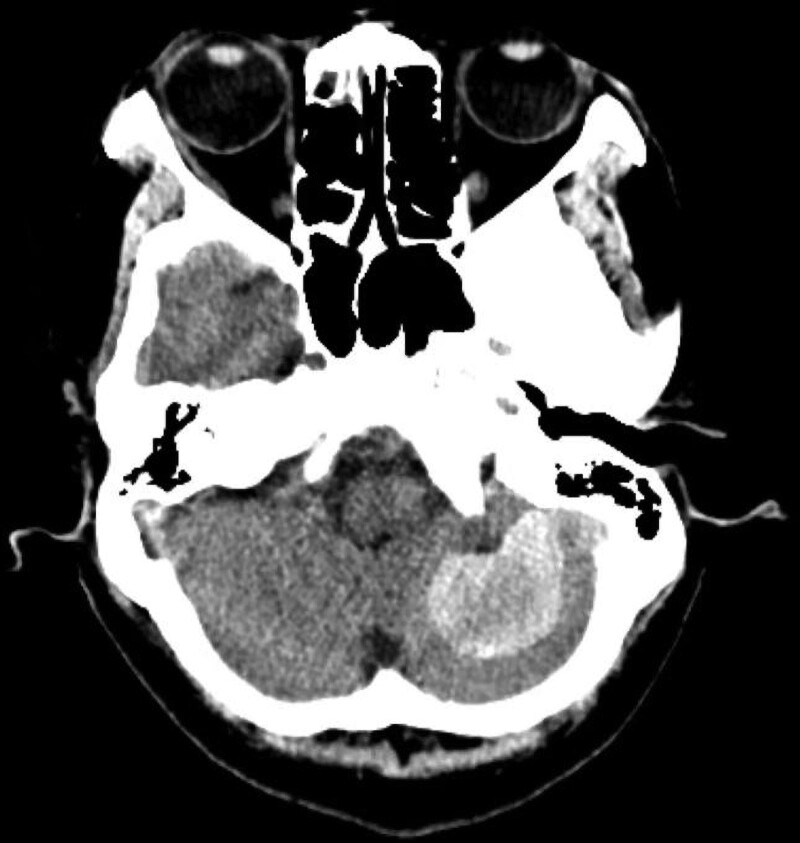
Axial head CT revealed a 3.4 cm × 2.5 cm well-defined slightly hyperdense mass without peritumoral edema in the left cerebellar hemisphere. CT = computed tomography.
